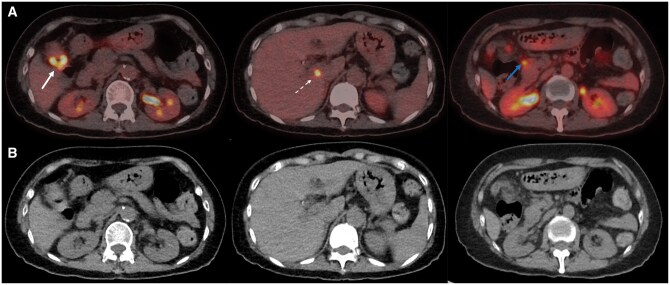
(A) Axial fused [18F]-FDG PET/CT abdomen, and (B) axial low-dose CT. The images demonstrate intense FDG uptake within the hepatic flexure (solid white arrow) as well as intense hypermetabolic activity in the caudate of the liver (broken white arrows), and moderate uptake in an adjacent pericolic lymph node (solid blue arrow).
